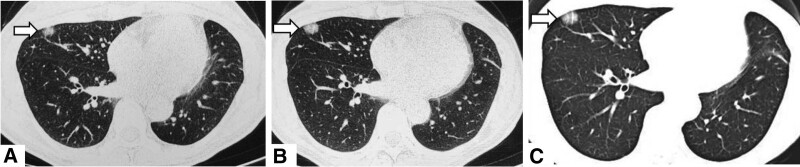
(A) Chest CT showed: the right lung middle lobe lateral segment subpleural see about 7.0 mm in diameter in the right middle lobe circular ground glass nodules, the edge of the lesion is unclear. (B) After 2 months, chest CT showed: subpleural section of lateral segment of middle lobe of right lung 10.0 × 13.0 mm-like round ground glass nodule. The lesion was slightly more dense and slightly enlarged in size compared with the previous one. (C) 3D imaging CT of both lungs showed: increased texture of both lungs, a mixed ground glass nodule shadow is seen in the outer segment of the middle lobe of the right lung, about 1.5 cm in size × 1.1 cm. CT = computed tomography.
